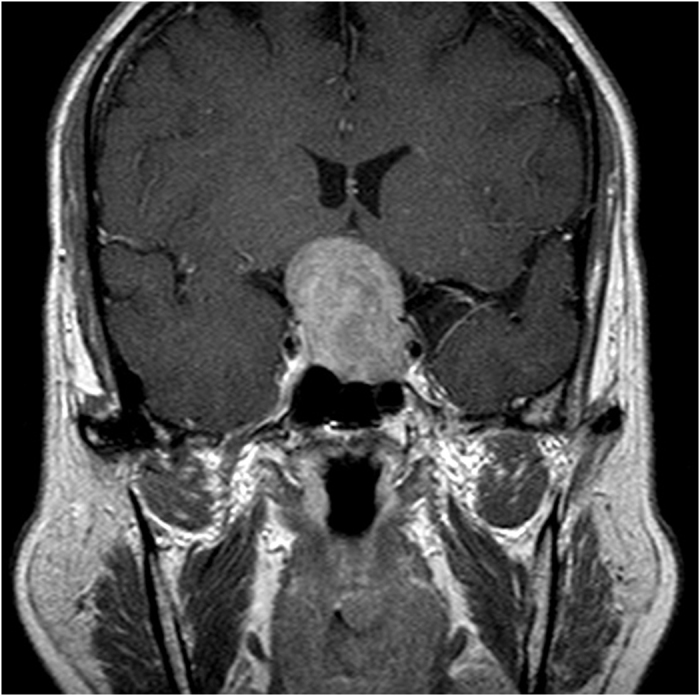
Preoperative MRI of a 62-year-old female patient revealed a typical NFPA with a regularly shaped tumor.
